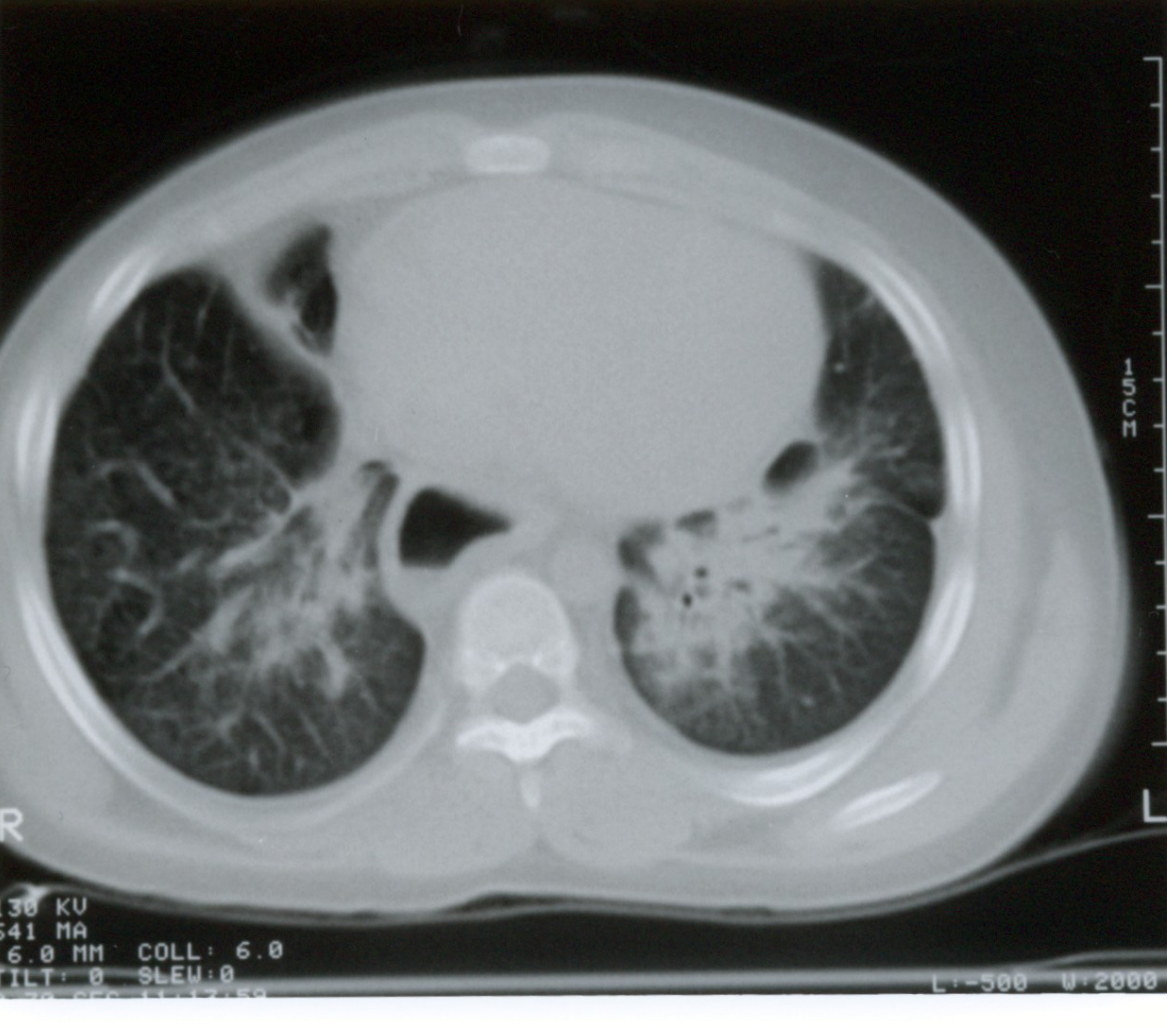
CT scan of chest showing bilateral lung opacification with septal thickening and esophageal dilatation.
